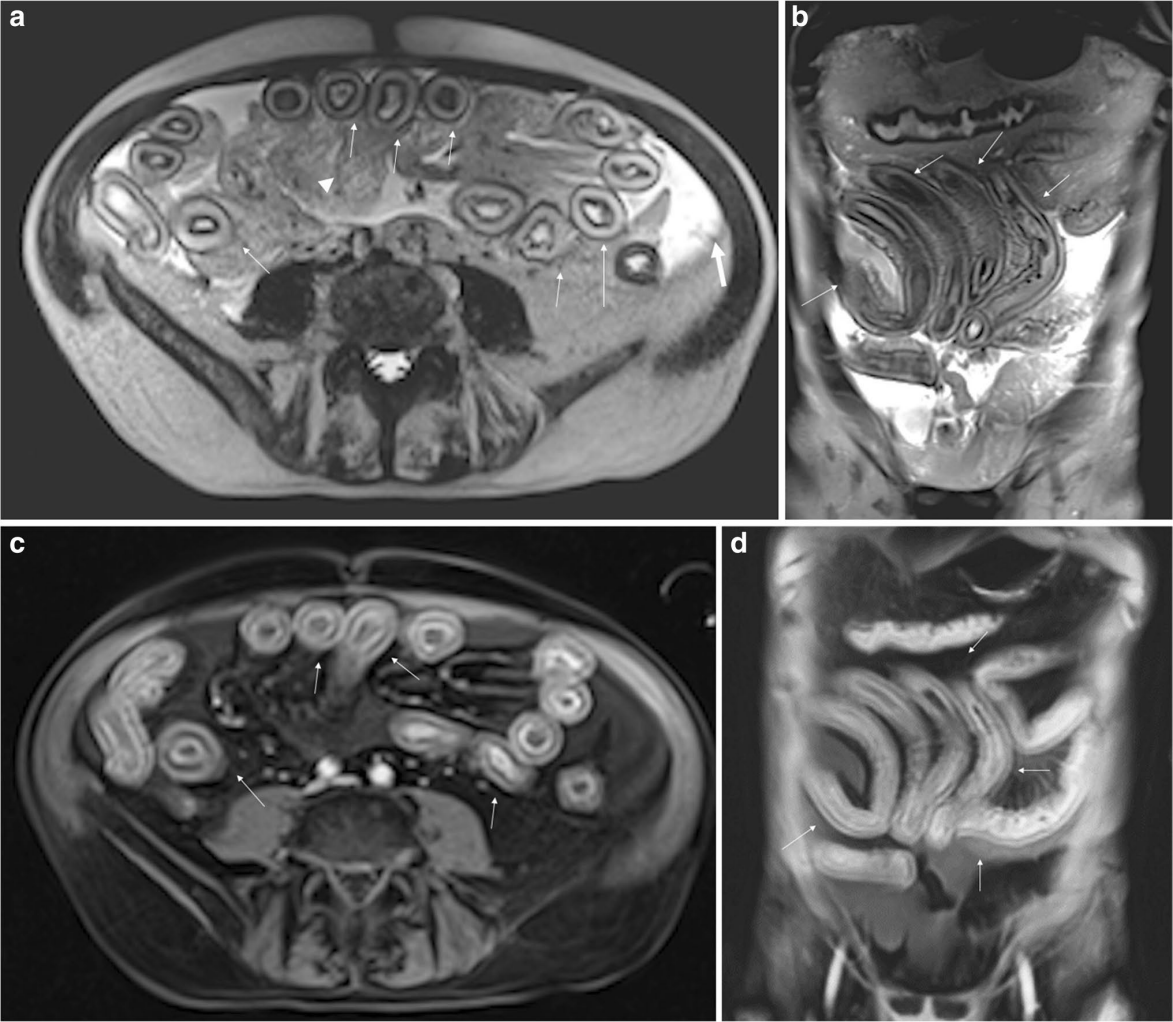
Acute intestinal GVHD in a 56-year-old man, Glucksberg grade IV (severe disease, high mortality risk). The MRI severity score is very high (24) and classified as “severe” disease (high mortality risk). The entire small intestine is involved. The patient died a few days later. a (T2-weighted axial image) and b (T2-weighted coronal image) show diffuse concentric *wall thickening and marked stratification of the intestinal wall* and *wall edema (thin arrows)*, *ascites* (thick arrows), and edema of retroperitoneal tissues and mesenteric fatty tissue (arrowhead). c (T1-weighted gadolinium-enhanced axial image) and d (T1-weighted gadolinium-enhanced coronal image) show severe *wall thickening with marked wall contrast enhancement and stratification* (thin arrows). The coronal plane clearly shows the *stiffness of the involved intestinal loops. Intestinal stiffness*: intended as a continuous diffuse and circumferential intestinal wall edema (continuous wall thickening) which causes a serpiginous and rigid appearance of the involved bowel, showing smooth rather than sharp angles
